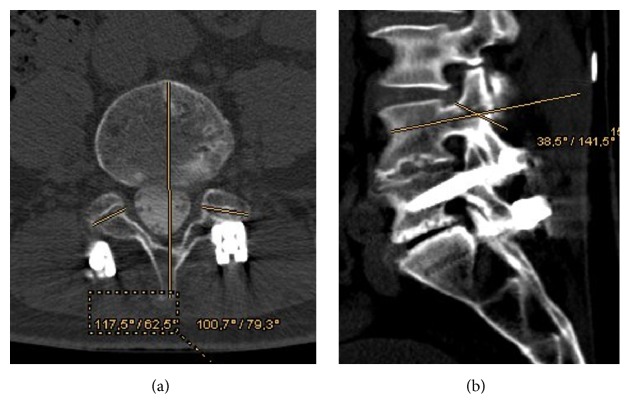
CT-scan of patient in Figure 5 with posterior lumbar interbody fusion in L5/S1 and ASD in L4/5. (a) Axial slice with facet tropism (17°) and (b) sagittal slice with laminar horizontalization of 141,5°.
